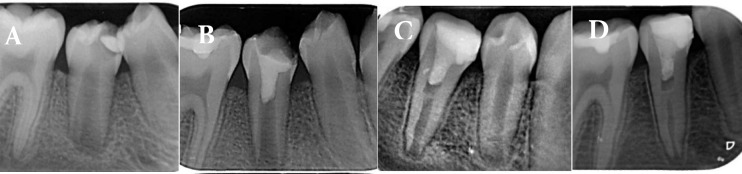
Preoperative radiography, B) Six-month follow-up after revascularization, C) Twelve-month follow-up, D) Two-year follow-up
